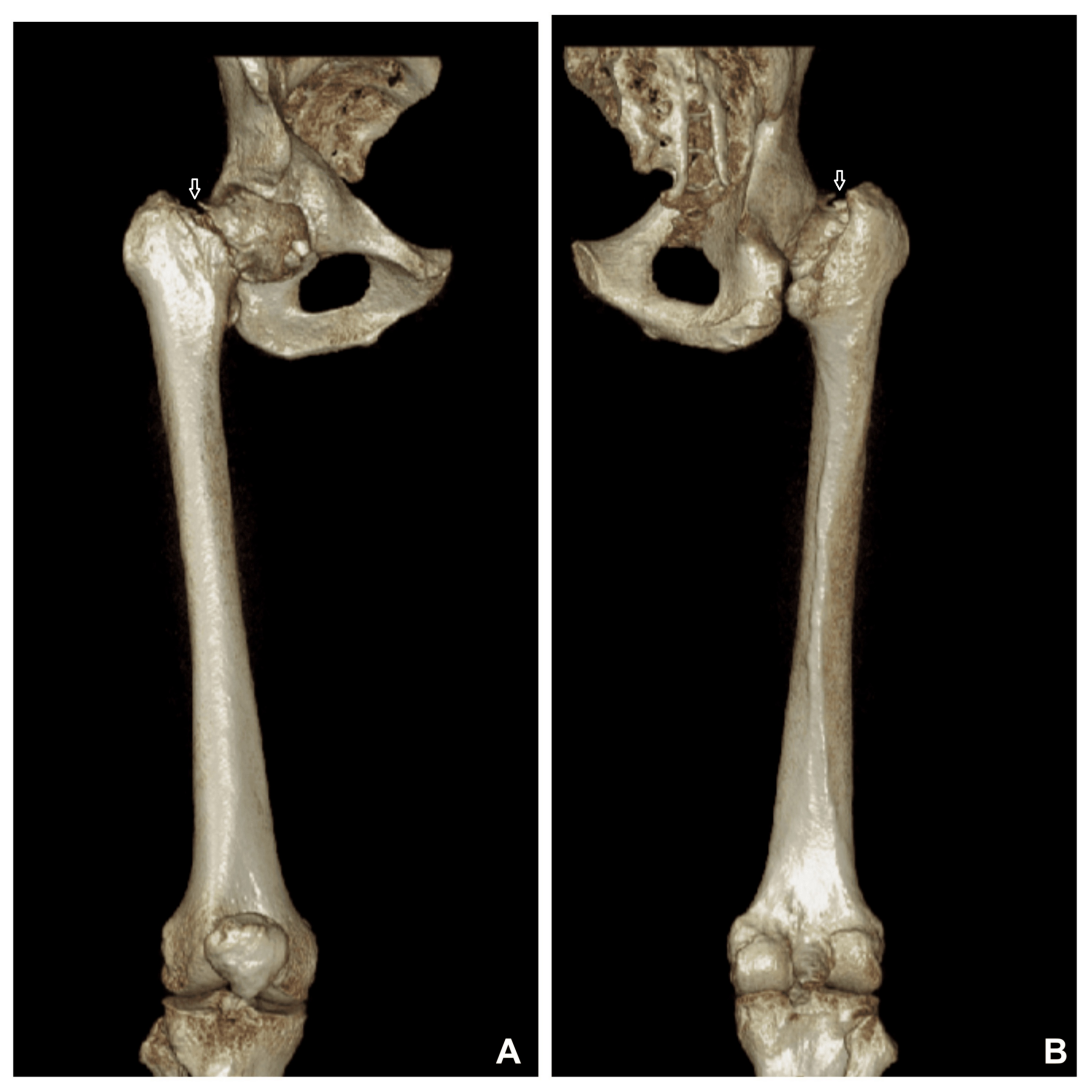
CT scan (3D bone window) showing basicervical fracture (white arrows) of the right femoral neck.
